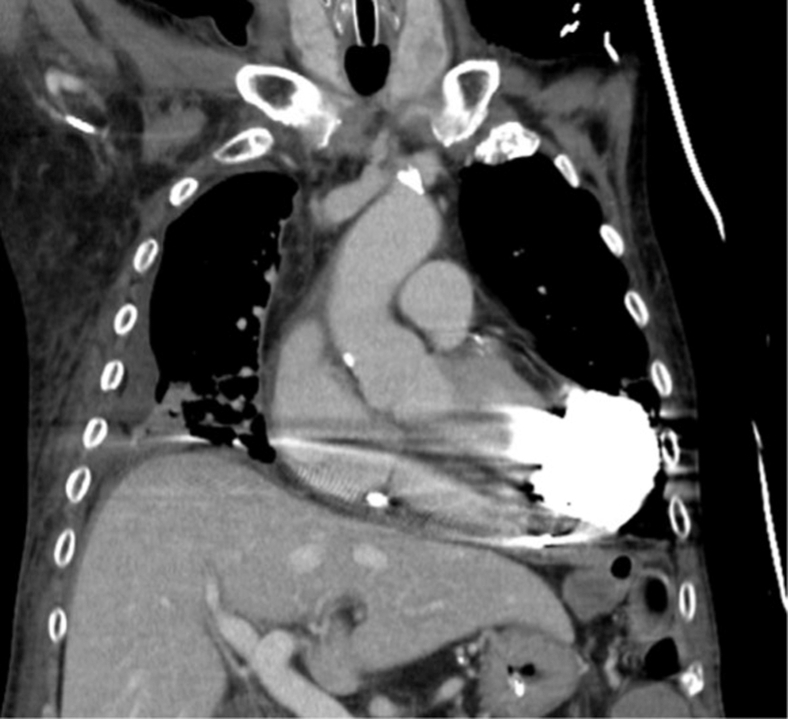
HeartMate 3 was implanted as a salvage strategy for recurrent left ventricular free-wall rupture. Follow-up contrast-enhanced computed tomography confirmed appropriate device positioning for left ventricular decompression.
